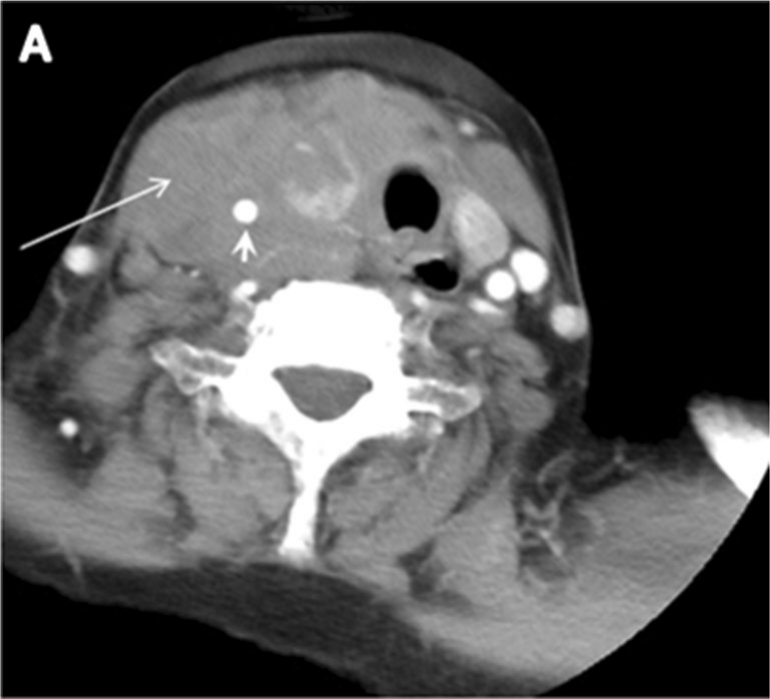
Diffuse thyroid large B-cell lymphoma in 79-year-old female patient. a Axial enhanced neck CT scan demonstrates a homogeneously hypodense and minimally enhancing large right thyroid solid mass (*long white arrow*) extending into the thyroid isthmus. It is encasing the right carotid artery (*short white arrow*) and displacing of the trachea and oesophagus to the left side
